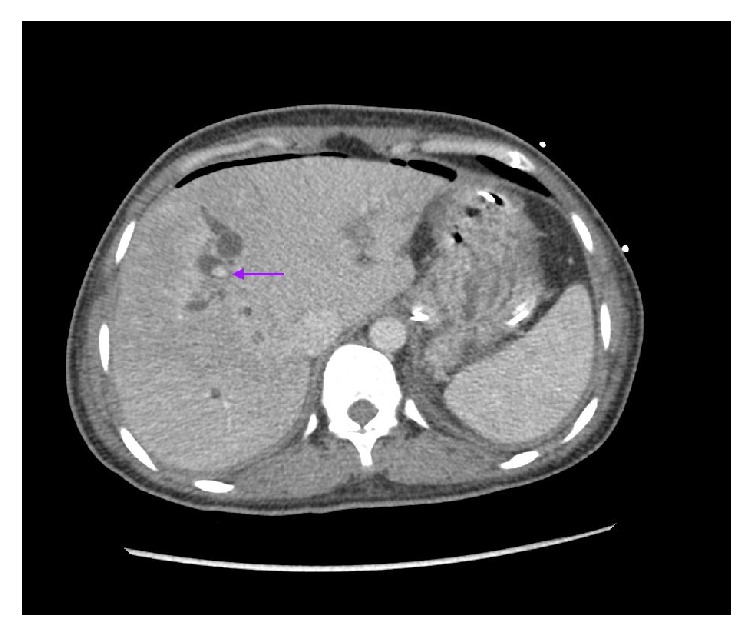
CT scan showing calcified stones within dilated intrahepatic ducts in the left lobe of the liver.
